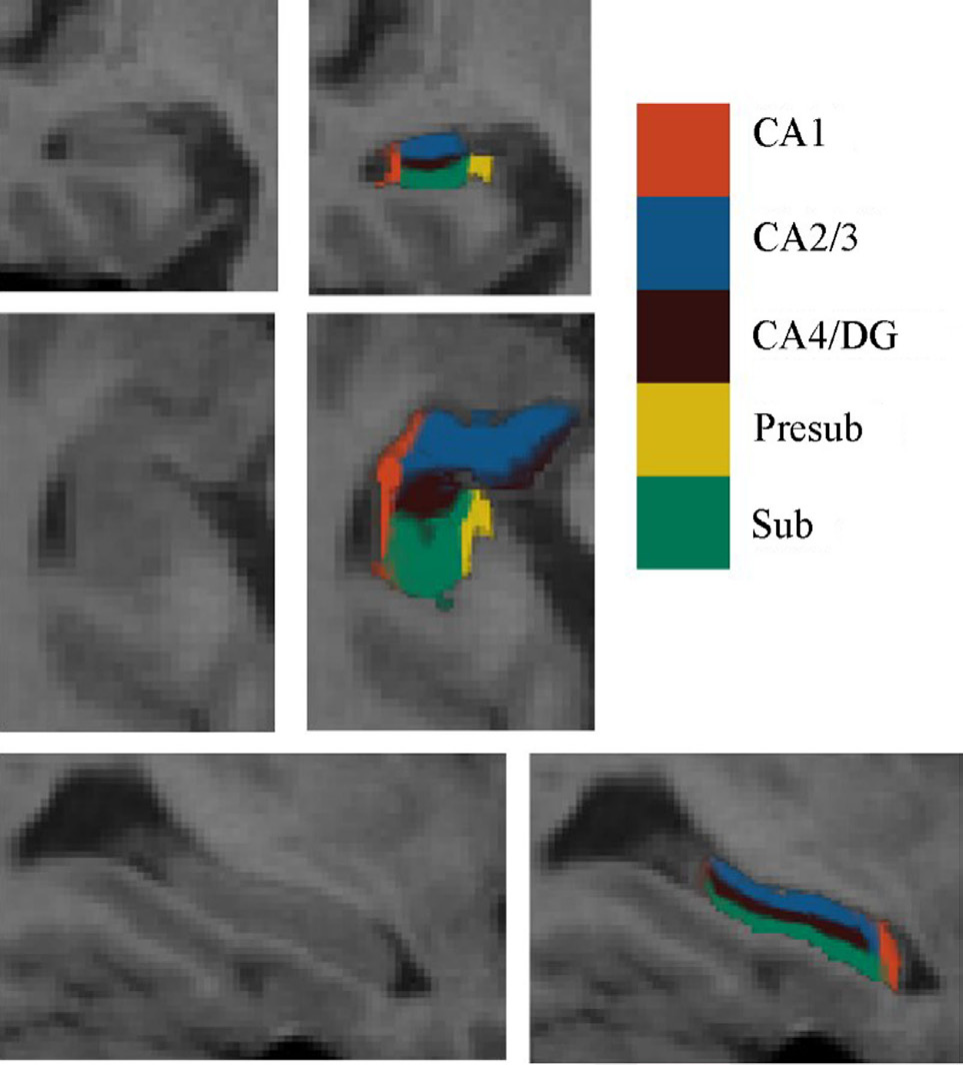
Right hippocampal subfield segmentation for one normal control subject. From left to right: cross-sectional slice of an MRI scan and corresponding automated segmentation of five subfields. Abbreviations: CA, cornu ammonis; DG, dentate gyrus; Presub, presubiculum; Sub, subiculum.
